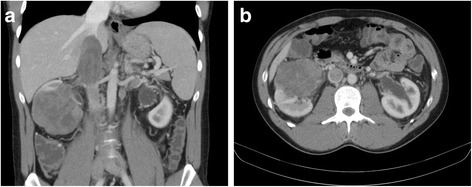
Abdominal CT scan at the time of diagnosis. Right kidney tumor with inferior vena cava tumor thrombus extending to the hepatic veins’ level. a Coronal plane. b Transverse plane
